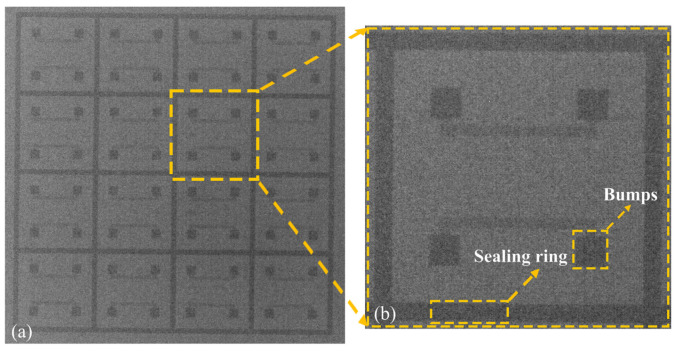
X-ray image. (a) Overall SEM image of the chip. (b) Enlarged view of a single unit.
